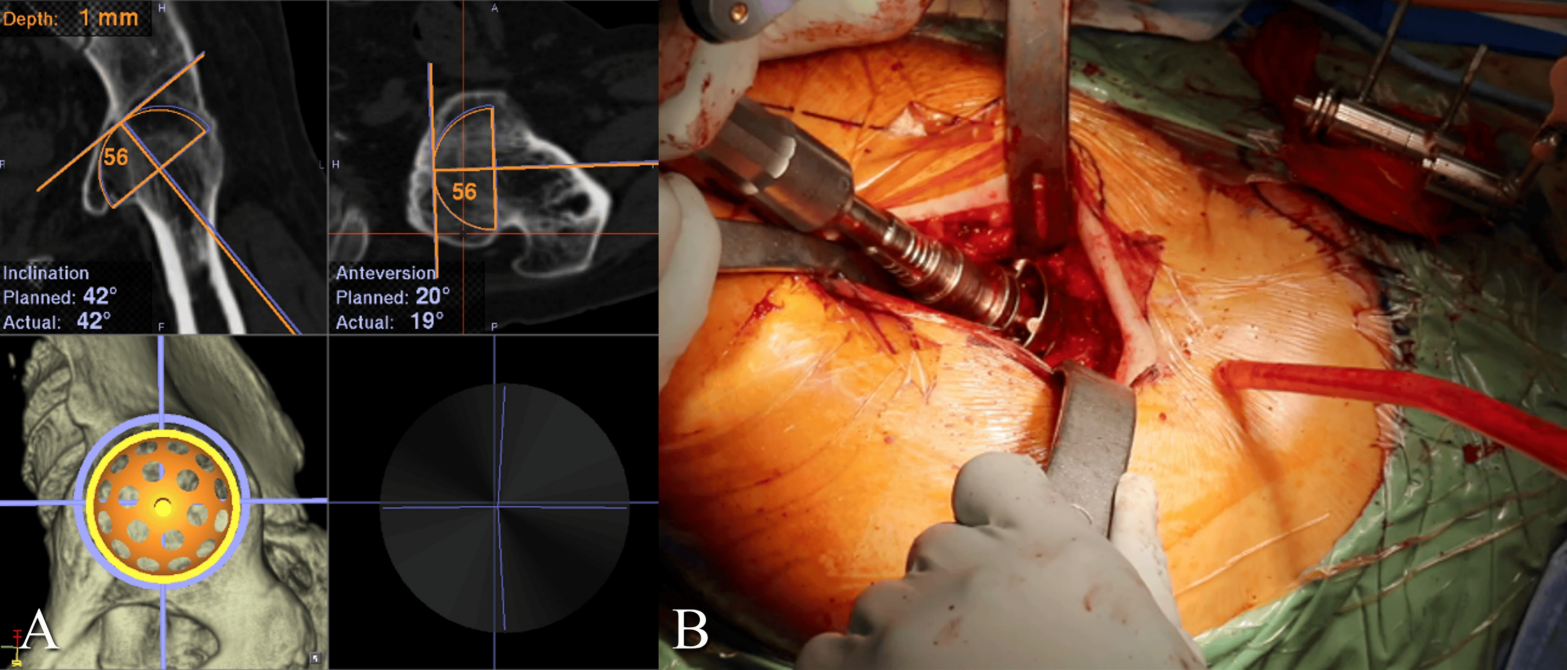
Computed tomography navigation images obtained during the operation for Case 1. A. The image of navigation during the reaming of acetabular reaming. B. The image during the reaming of acetabular reaming.
